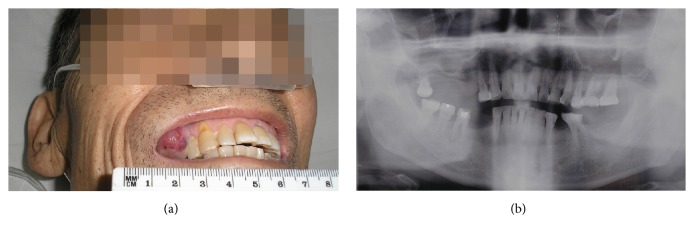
The gingival tumor and jawbone images. (a) A reddish gingival tumor of soft texture, clear margin, and central mucosal ulceration on the right upper maxillary. (b) Radiography without local bony destruction.
